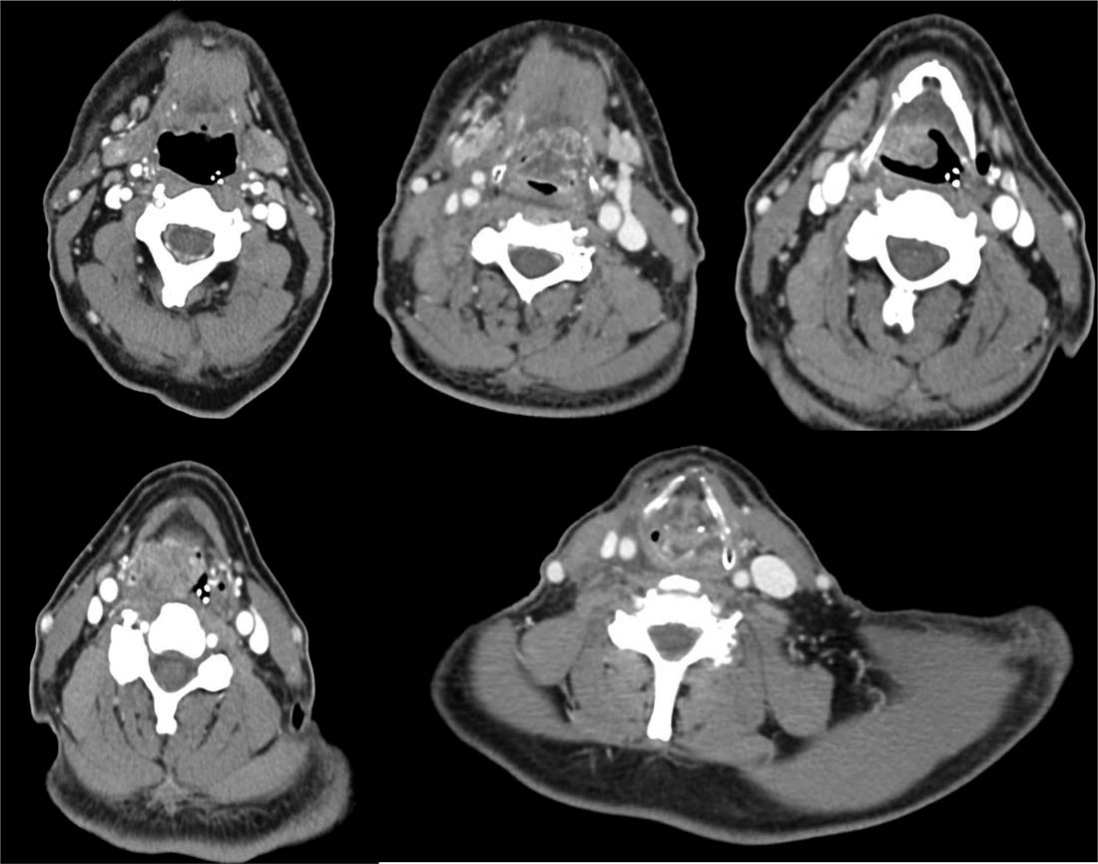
A contrast-enhanced CT scan of the head and neck, in coronal view, revealed a heterogeneous mass in the right supraglottic region, involving the aryepiglottic fold and piriform sinus with full-thickness infiltration. The lesion extended to the paralaryngeal and parapharyngeal spaces, causing obliteration of adipose planes and thyroid cartilage invasion and severe airway reduction.
